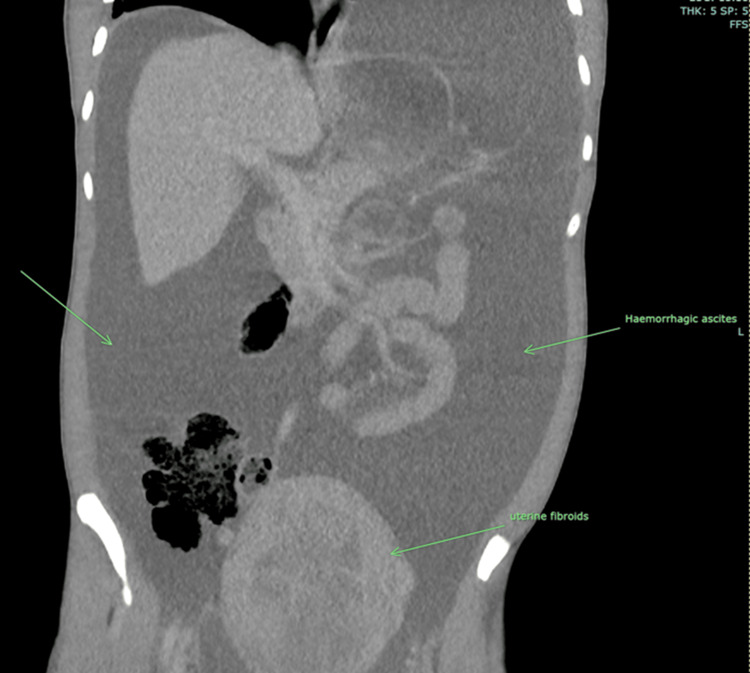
CT image demonstrating ascites and fibroid uterus in Case 1
